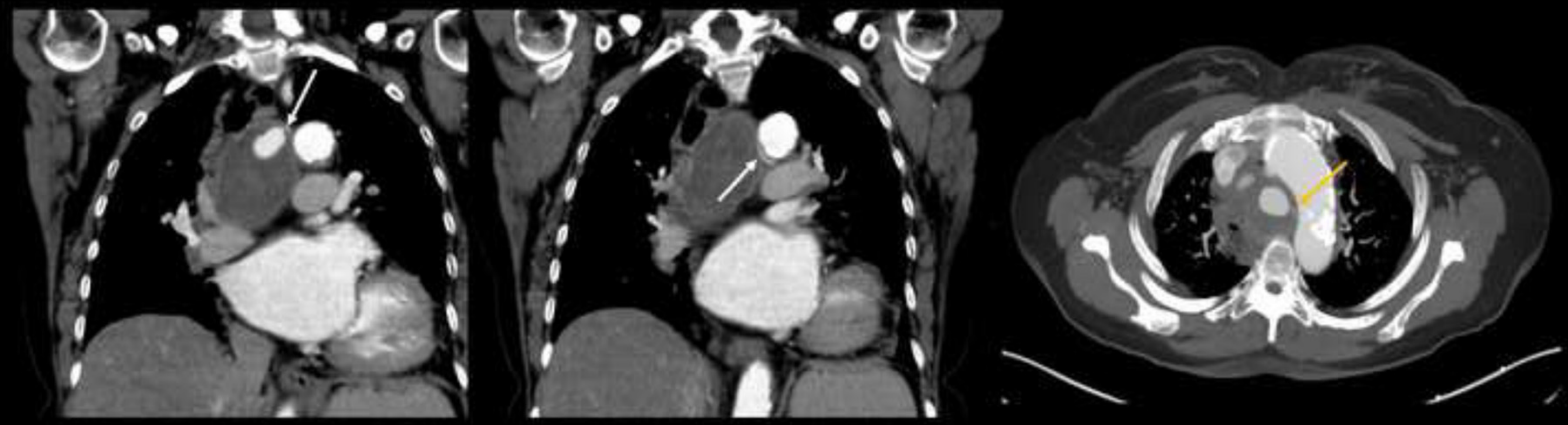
CT angiogram shows peripherally thrombosed pseudoaneurysm, arising from right bronchial artery (arrow).
